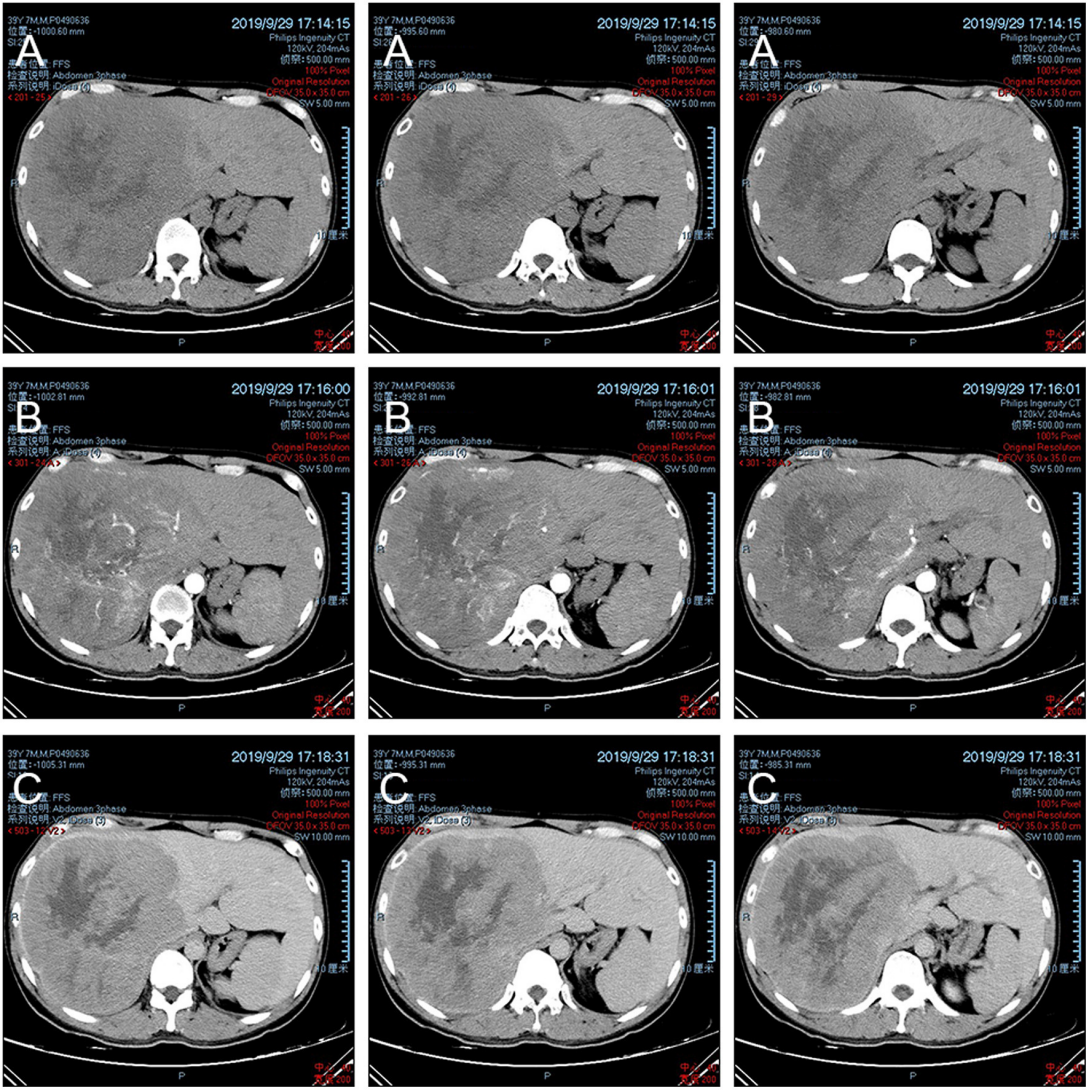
CT images. (A) Plain scan; (B) arterial phase; and (C) portal venous phase.
